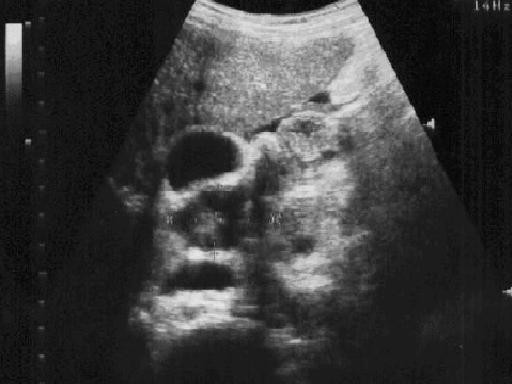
Longitudinal sonogram of liver showing a large retroperitoneal lymph node adjacent to the gall bladder in a 27 year-old asymptomatic HIV+ patient.
